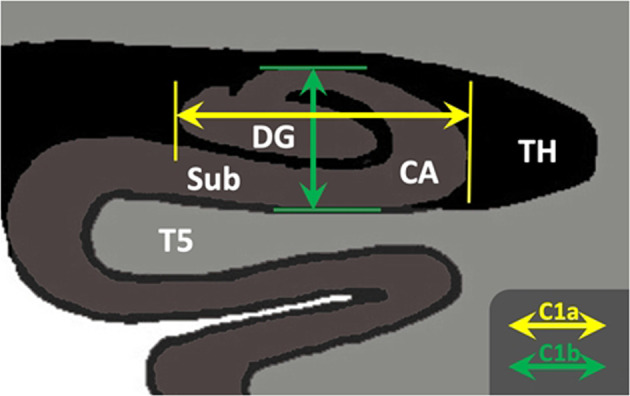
Anatomical criteria used to define hippocampal roundness in a coronal MRI view. The horizontal arrow (C1a) goes from the medial part of the dentate gyrus (DG) to the lateral part of the hippocampus. The vertical arrow (C1b) goes from the bottom to the top part of the cornu Ammonis (CA). When C1a > C1b, the hippocampus is considered “flat”, and round/oval otherwise (later called “nonflat”). Sb subiculum, TH temporal horn.
